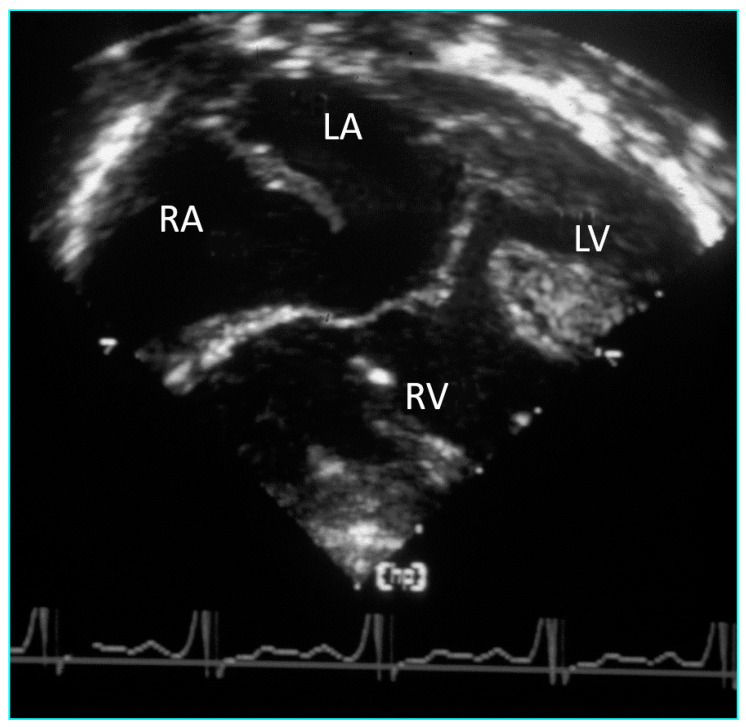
Echocardiographic four chamber section from a heart with complete AVSD, hypoplastic left ventricle and the morphological criteria for the double inlet right ventricle.
